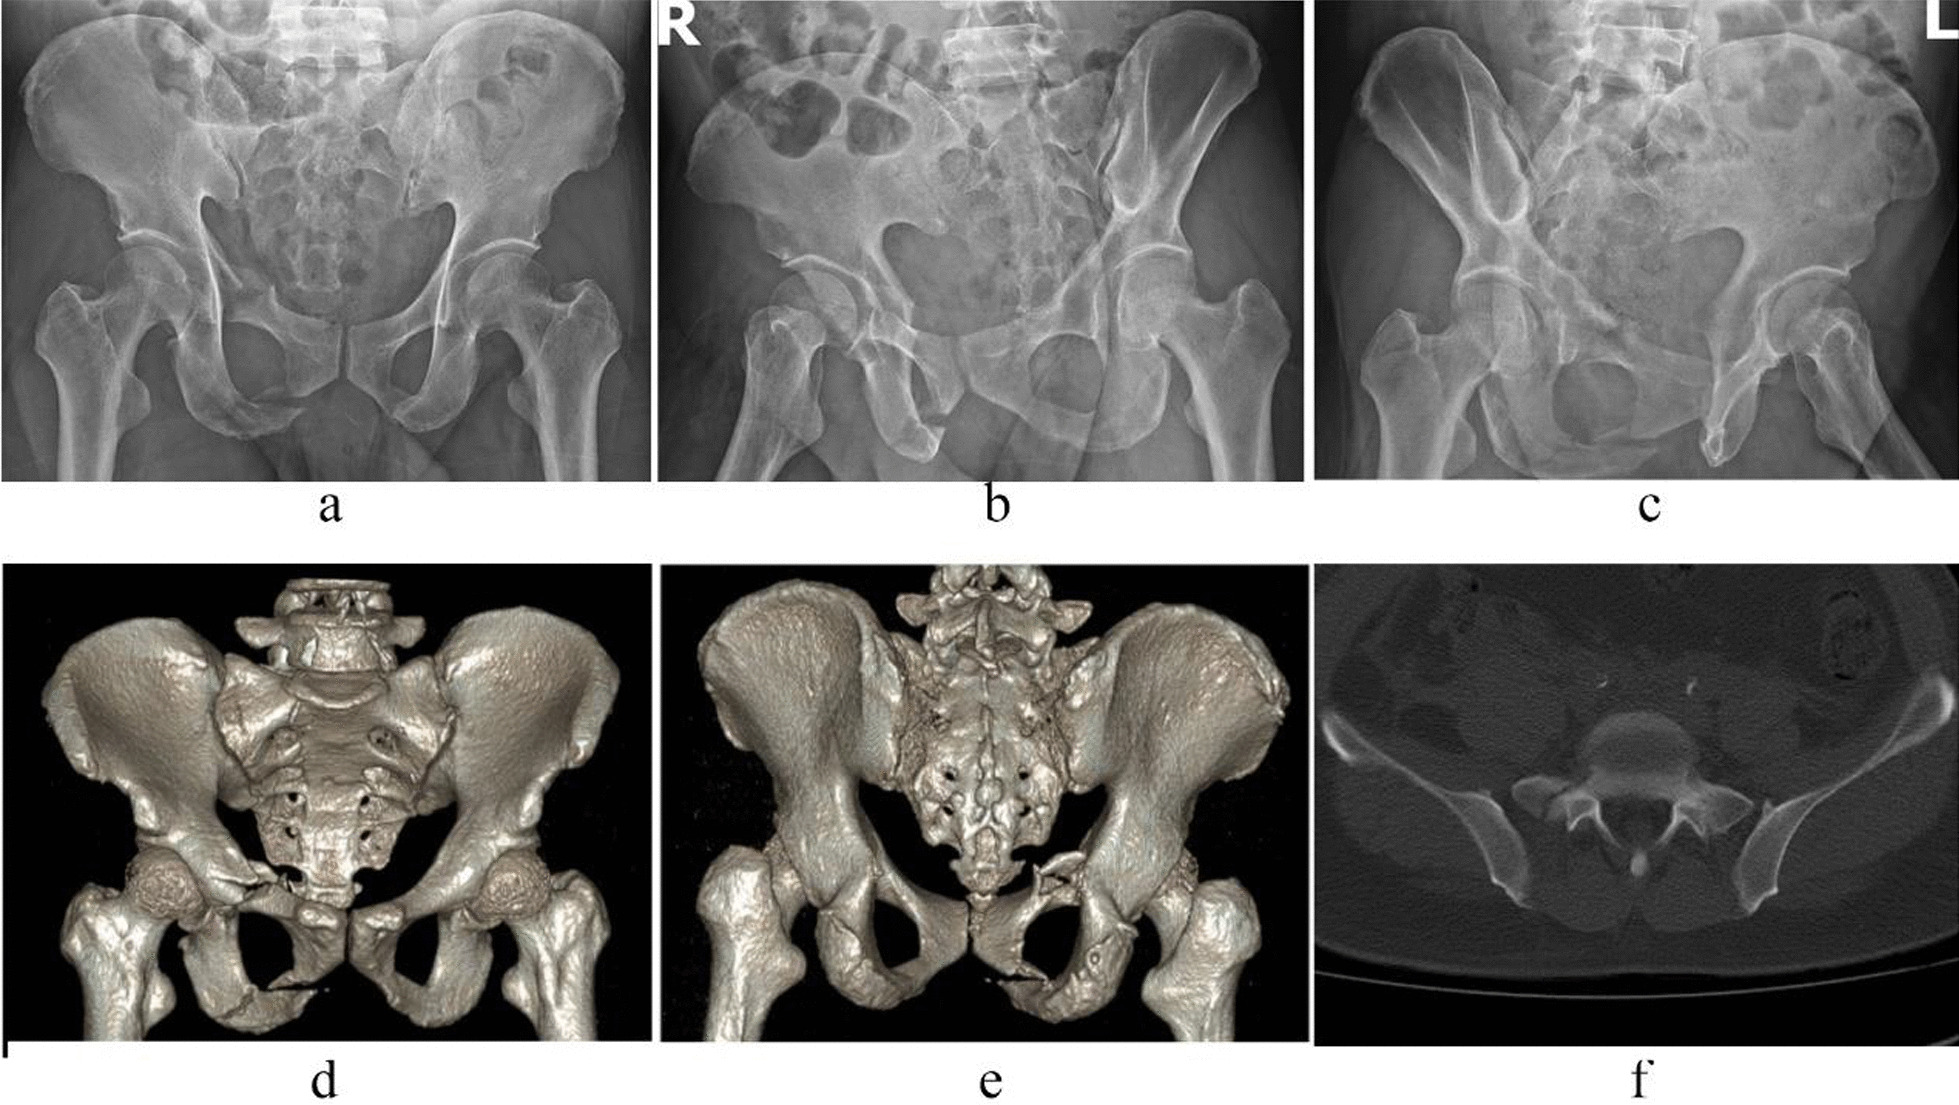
Preoperative X-ray and CT images of case 1. (a Pelvic anteroposterior X-ray image, b iliac oblique position X-ray image, c obturator oblique position X-ray image, d, e preoperative CT 3D reconstruction image, f CT horizontal slice image.)
